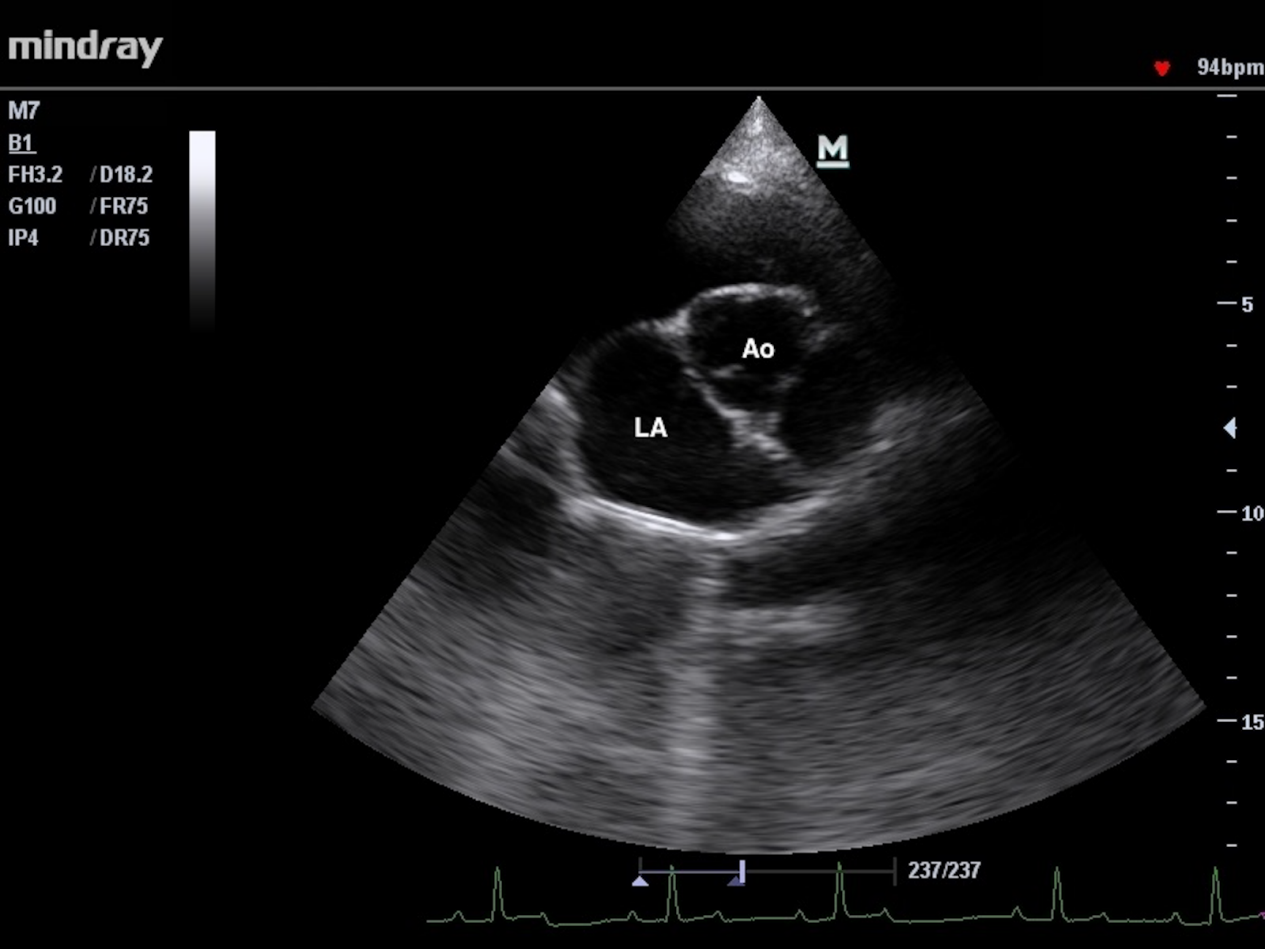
The right parasternal short-axis view of the heart base with aorta. LA- left atrium, Ao- aorta.
